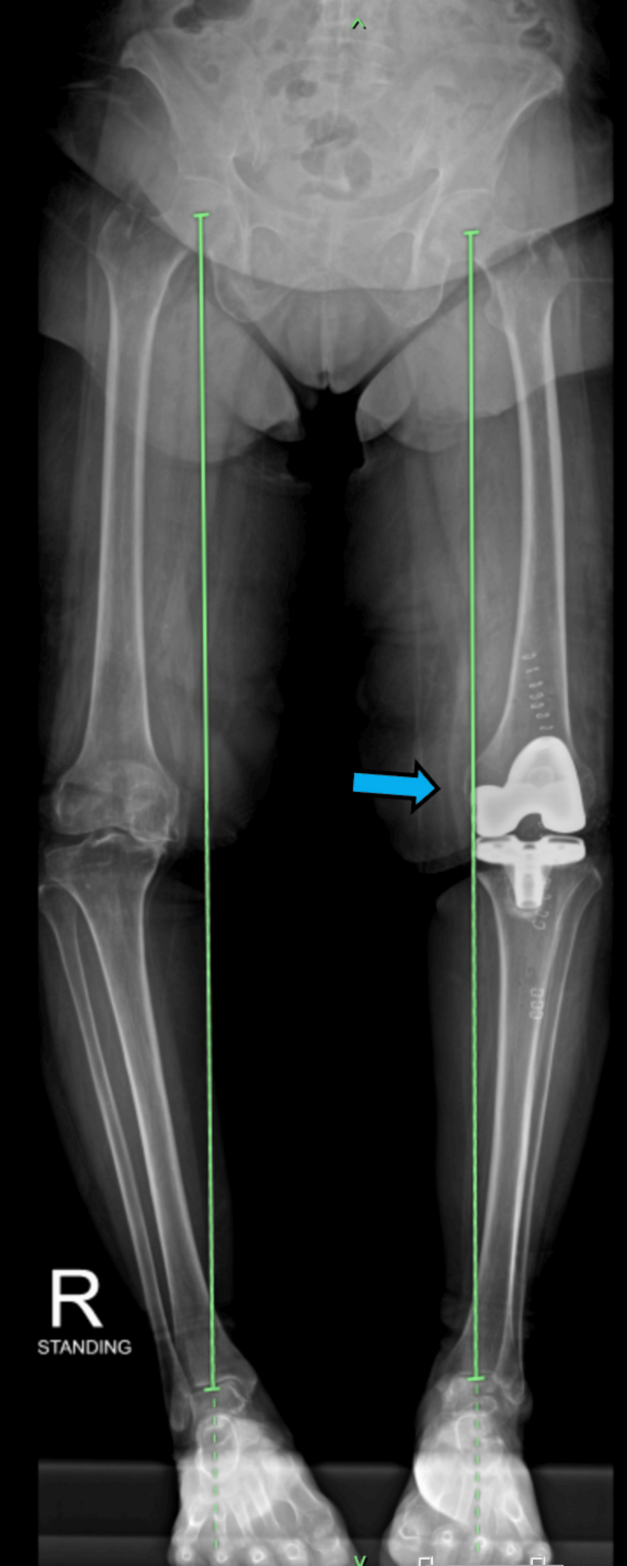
Plain X-ray of the bilateral lower limb showed implant in situ and the mechanical axis of the left lower limb improved after left total knee arthroplasty.
